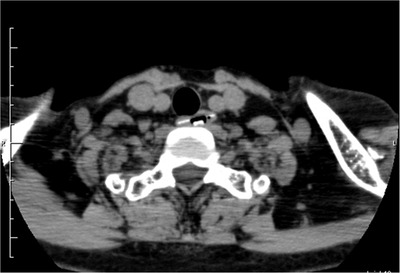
Cervicothoracic computed tomography reveals a 17 mm^2^ PTP sheet inside the cervical esophagus
